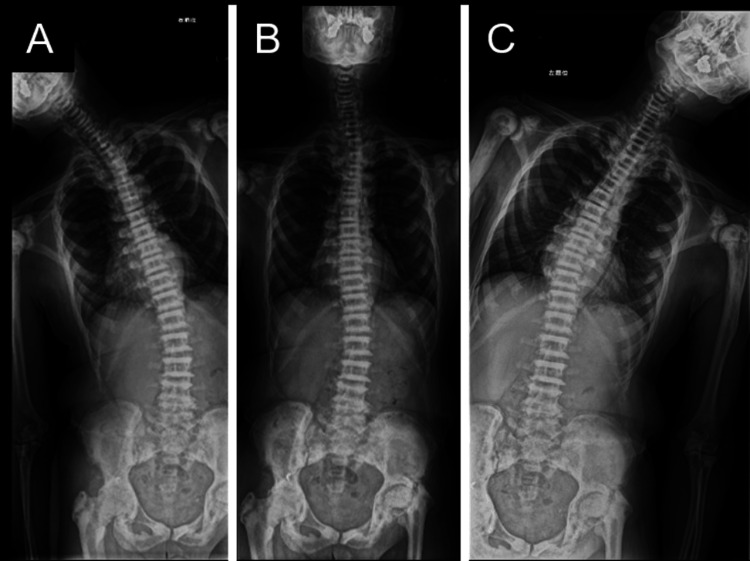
Preoperative X-ray images of the spine A, right lateral flexion; B, neutral; C, left lateral flexion Poor lateral flexion flexibility in the lumbar spine was indicated.
